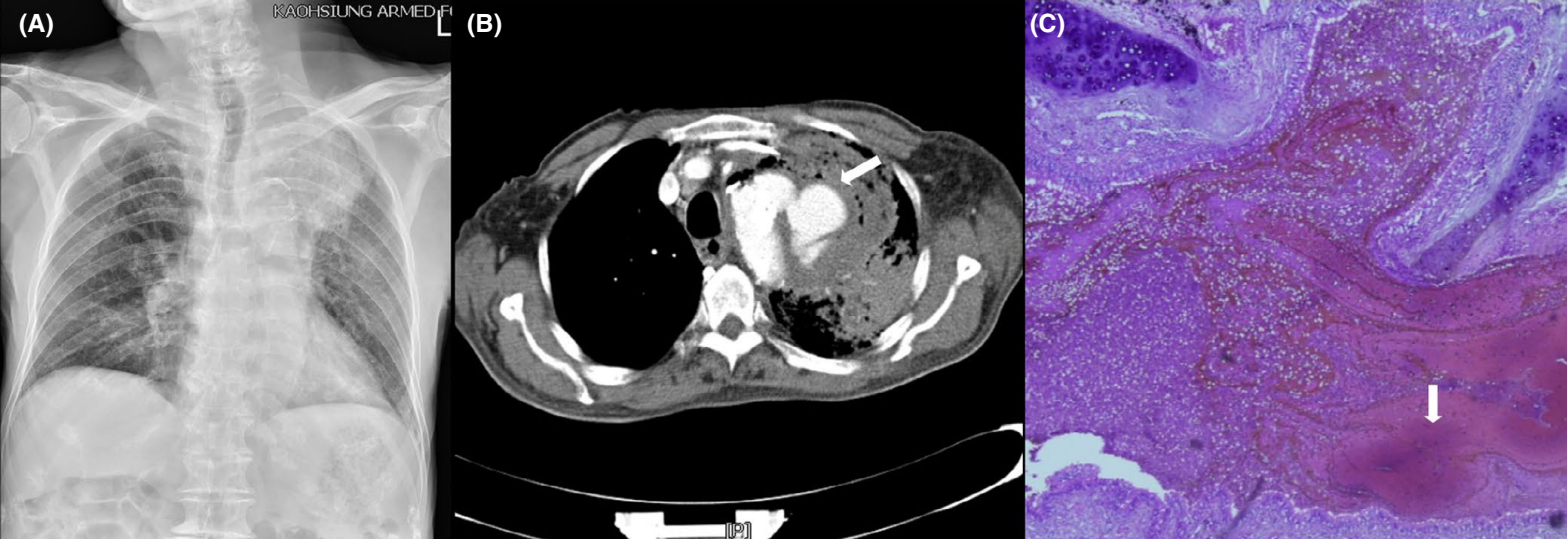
(A) Chest radiograph of an 87‐year‐old man obtained 3 h after presentation to the emergency department for survey of worsening hemoptysis. Evident progression of opacification over left upper lung was noted. (B) Computed tomography of chest was promptly performed, which demonstrated one ruptured thoracic aortic aneurysm (arrow). (C) Surgical pathology demonstrated whole‐layer inflammation of aortic wall and hemorrhage into lung parenchyma, causing hemobronchus and pulmonary hemorrhage (arrow)
